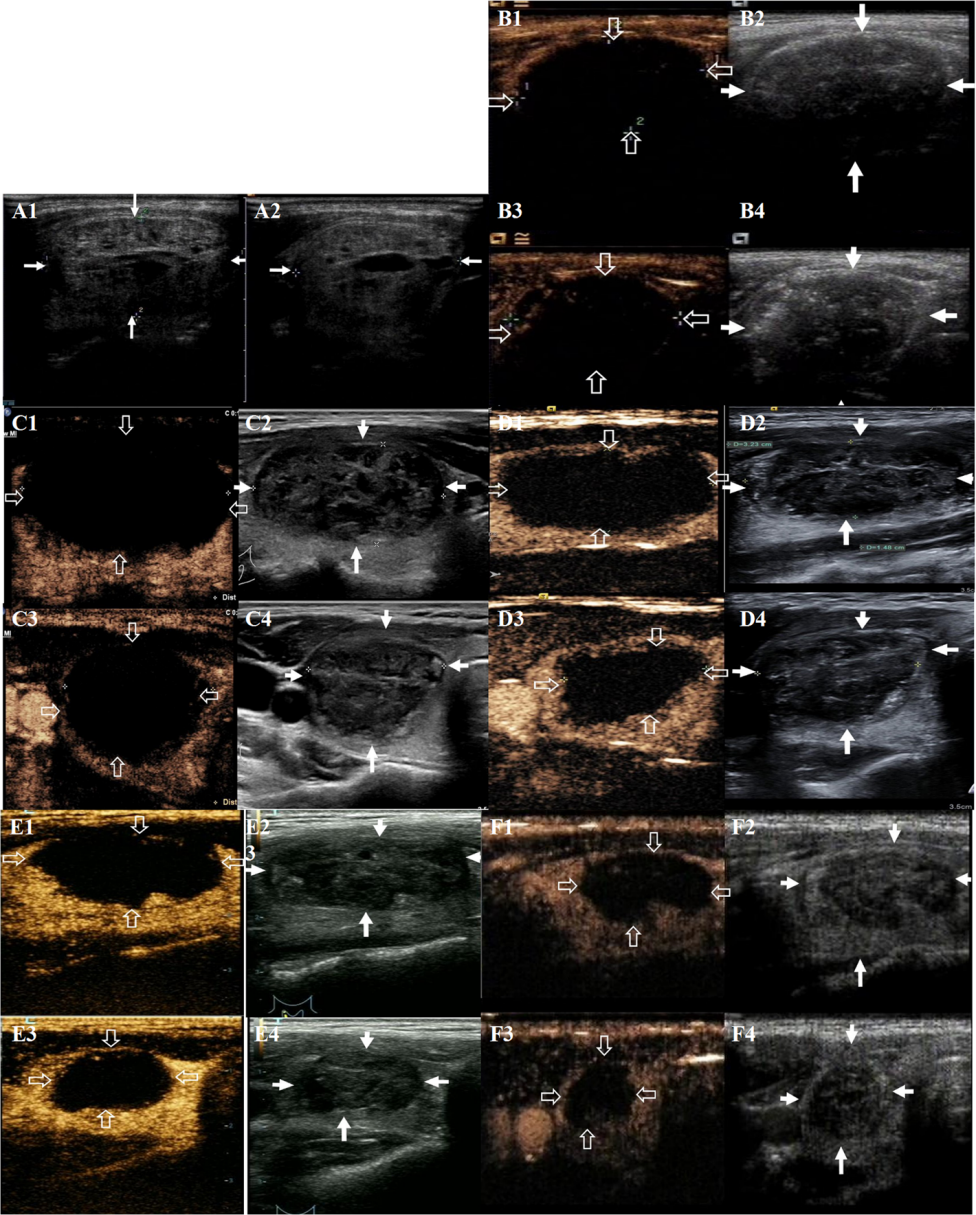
Preoperative and postoperative ultrasonography of one patient. A: preoperative ultrasonography (A1: longitudinal section of two-dimensional ultrasonography; A2: transverse section of two-dimensional ultrasonography); B: postoperative ultrasonography at 0 months (A1: longitudinal section of contrast-enhanced ultrasonography; A2: longitudinal section of two-dimensional ultrasonography; A3: transverse section of contrast-enhanced ultrasonography; A4: transverse section of two-dimensional ultrasonography); C: postoperative ultrasonography at 1 months (C1: longitudinal section of contrast-enhanced ultrasonography; C2: longitudinal section of two-dimensional ultrasonography; C3: transverse section of contrast-enhanced ultrasonography; C4: transverse section of two-dimensional ultrasonography); D: postoperative ultrasonography at 3 months (D1: longitudinal section of contrast-enhanced ultrasonography; D2: longitudinal section of two-dimensional ultrasonography; D3: transverse section of contrast-enhanced ultrasonography; D4: transverse section of two-dimensional ultrasonography); E: postoperative ultrasonography at 6 months (E1: longitudinal section of contrast-enhanced ultrasonography; E2: longitudinal section of two-dimensional ultrasonography; E3: transverse section of contrast-enhanced ultrasonography; E4: transverse section of two-dimensional ultrasonography); F: postoperative ultrasonography at 12 months (F1: longitudinal section of contrast-enhanced ultrasonography; F2: longitudinal section of two-dimensional ultrasonography; F3: transverse section of contrast-enhanced ultrasonography; F4: transverse section of two-dimensional ultrasonography)
